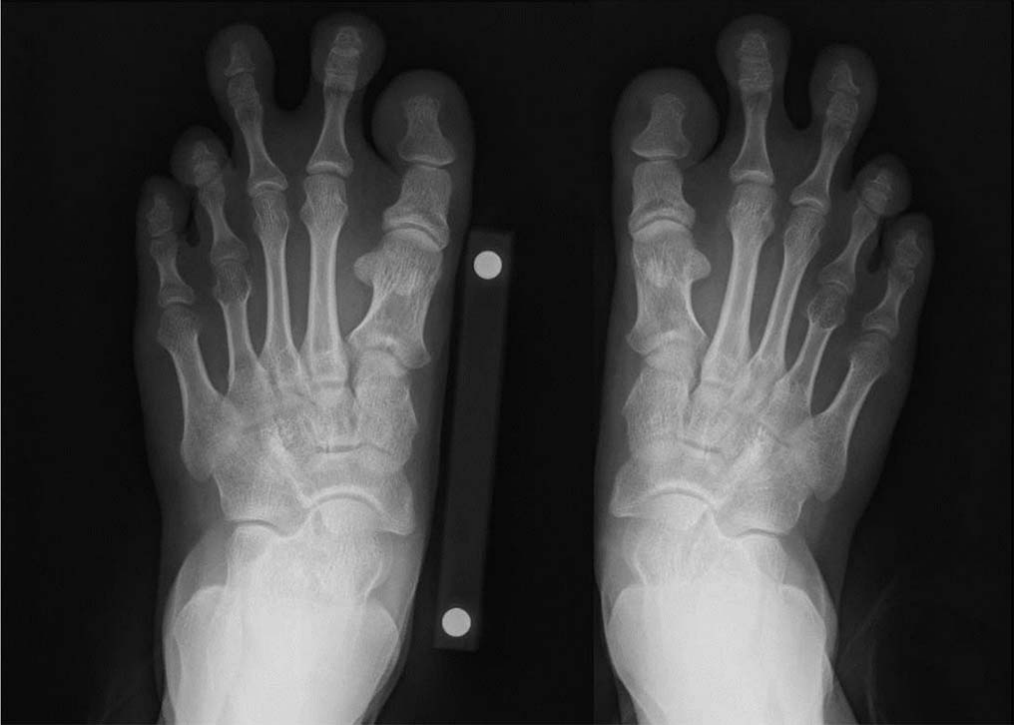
Preoperative anteroposterior radiograph of both feet, showing the short first, fourth, and fifth metatarsals, resulting in an irregular (non-smooth) curve (or parabola) that connects the 5 metatarsal heads.
